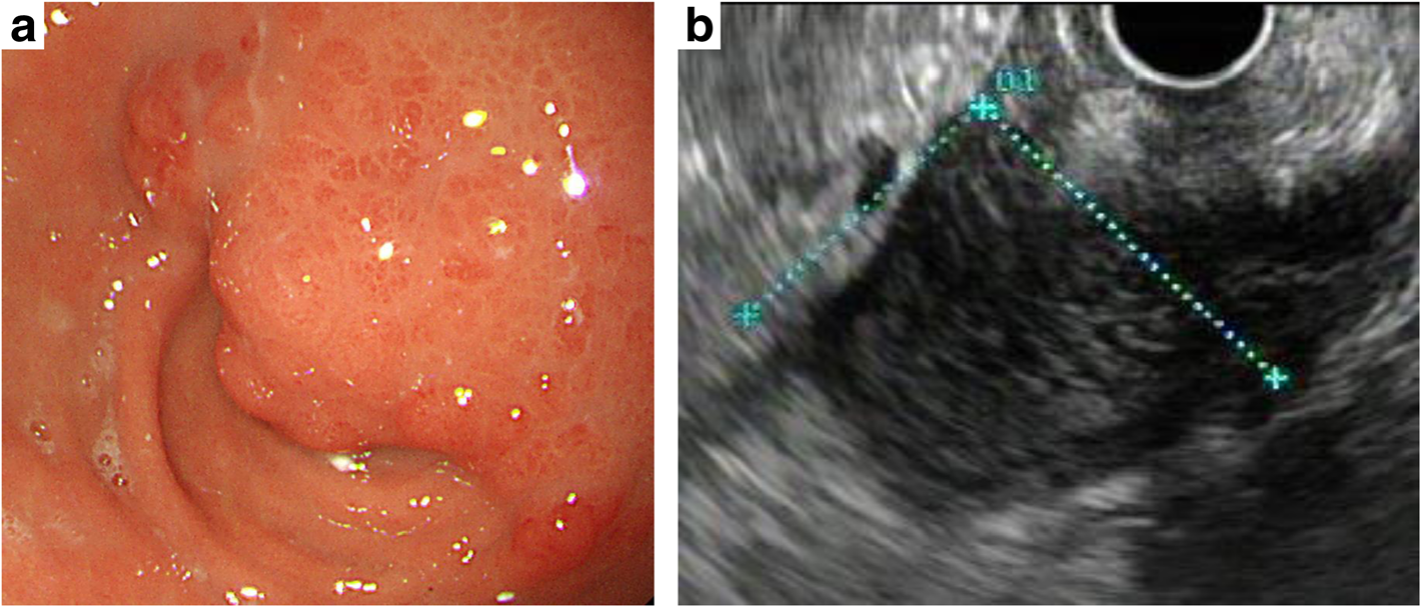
Endoscopic findings. a Upper endoscopy showing a submucosal tumor at the prepyloric antrum causing obstruction of the gastric outlet. b Endoscopic ultrasound showing a hypoechoic tumor located in the submucosal layer
